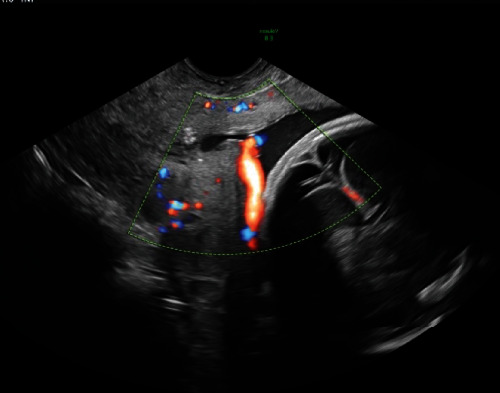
Transvaginal ultrasound image at 16 weeks showing vasa previa.
